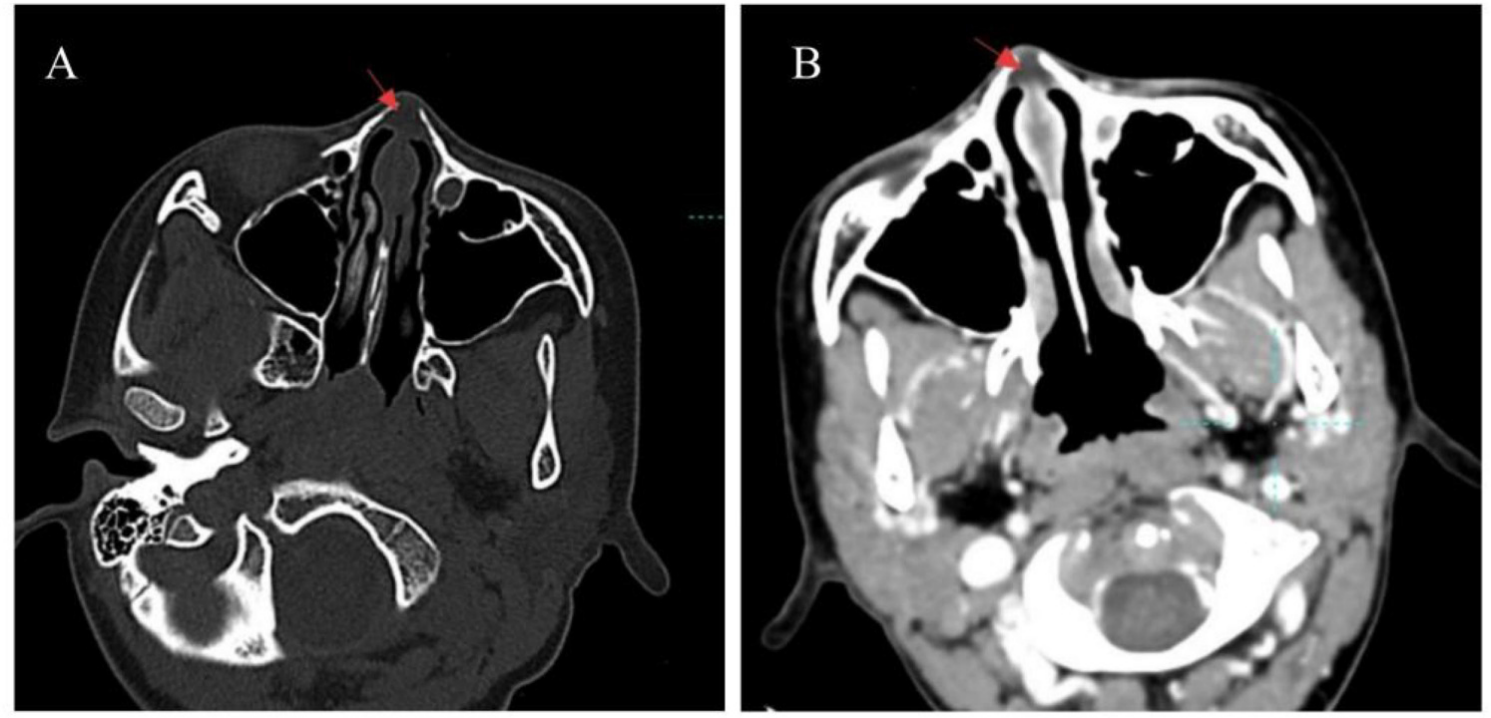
Representative CT image of nasal dermoid sinus cysts in pediatric. (A) A cystic low-density shadow in the middle of the nasal bone at the location indicated by the arrow, with a clear boundary. The bilateral nasal bones are compressed and displaced, presenting an arc-shaped indentation. (B) The area indicated by the arrow shows significant enhancement of the anterior soft tissue of the nasal septum after CT enhancement.
